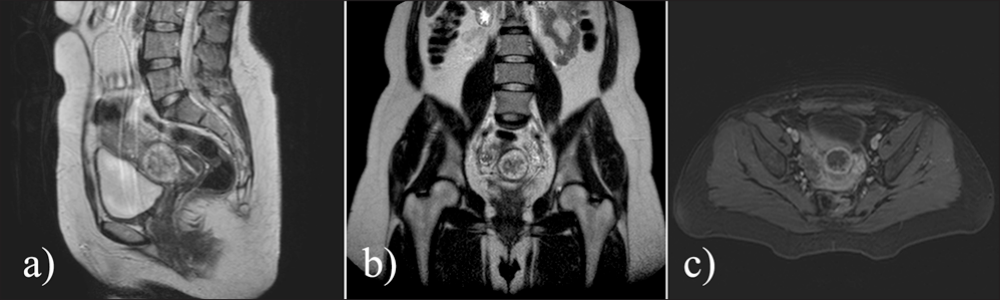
T2-weighted sagittal (a) and coronal (b) MR images demonstrate a peripherally hyperintense and centrally hypointense lesion located in the cesarean section scar of the uterus. The lesion demonstrated peripheral contrast-enhancement after gadolinium administration (c).
